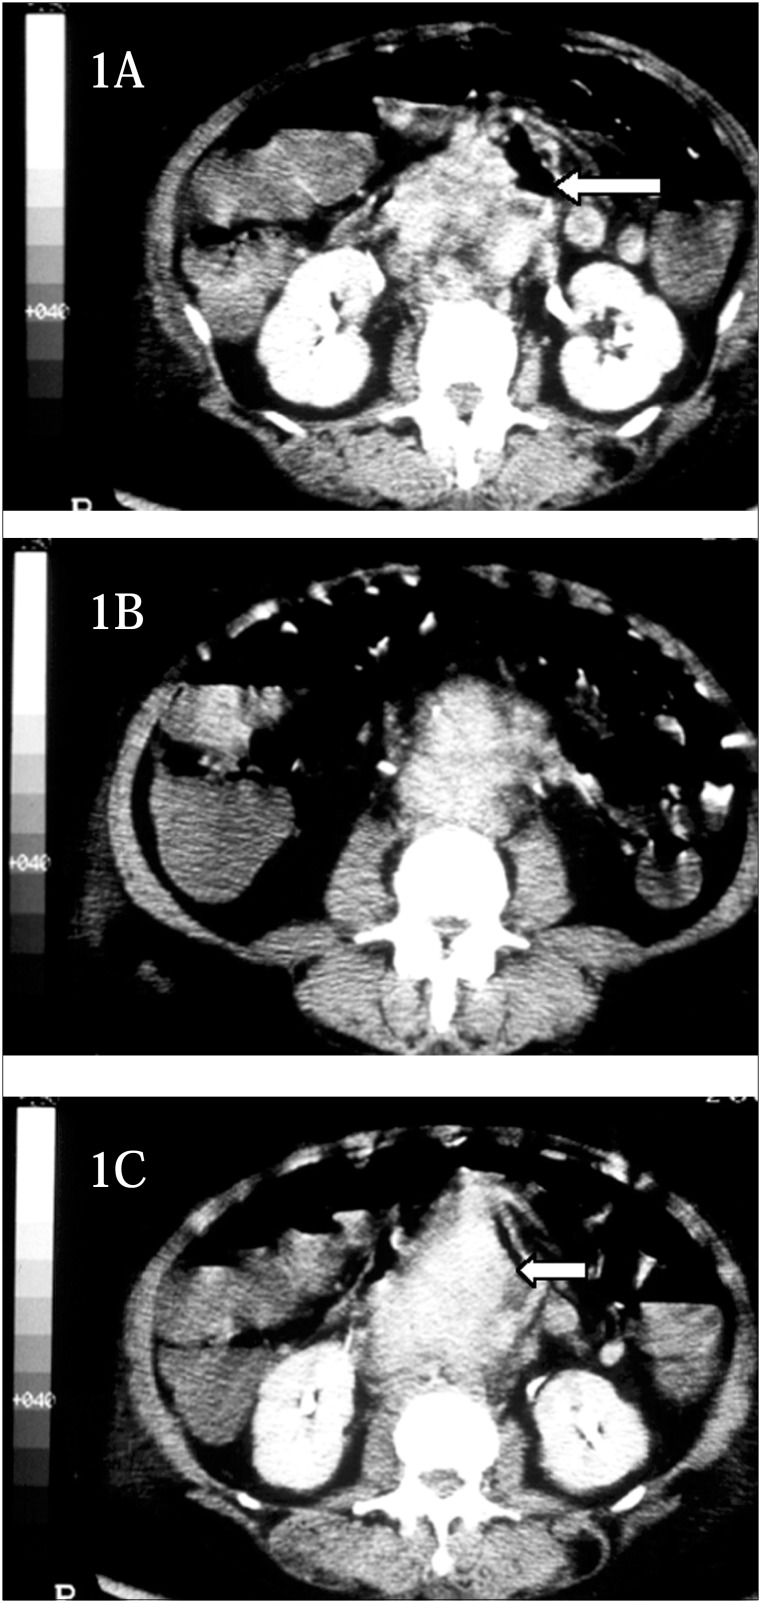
CT scan reveals a large pseudoaneurysm with air close to aortic wall (arrows).
